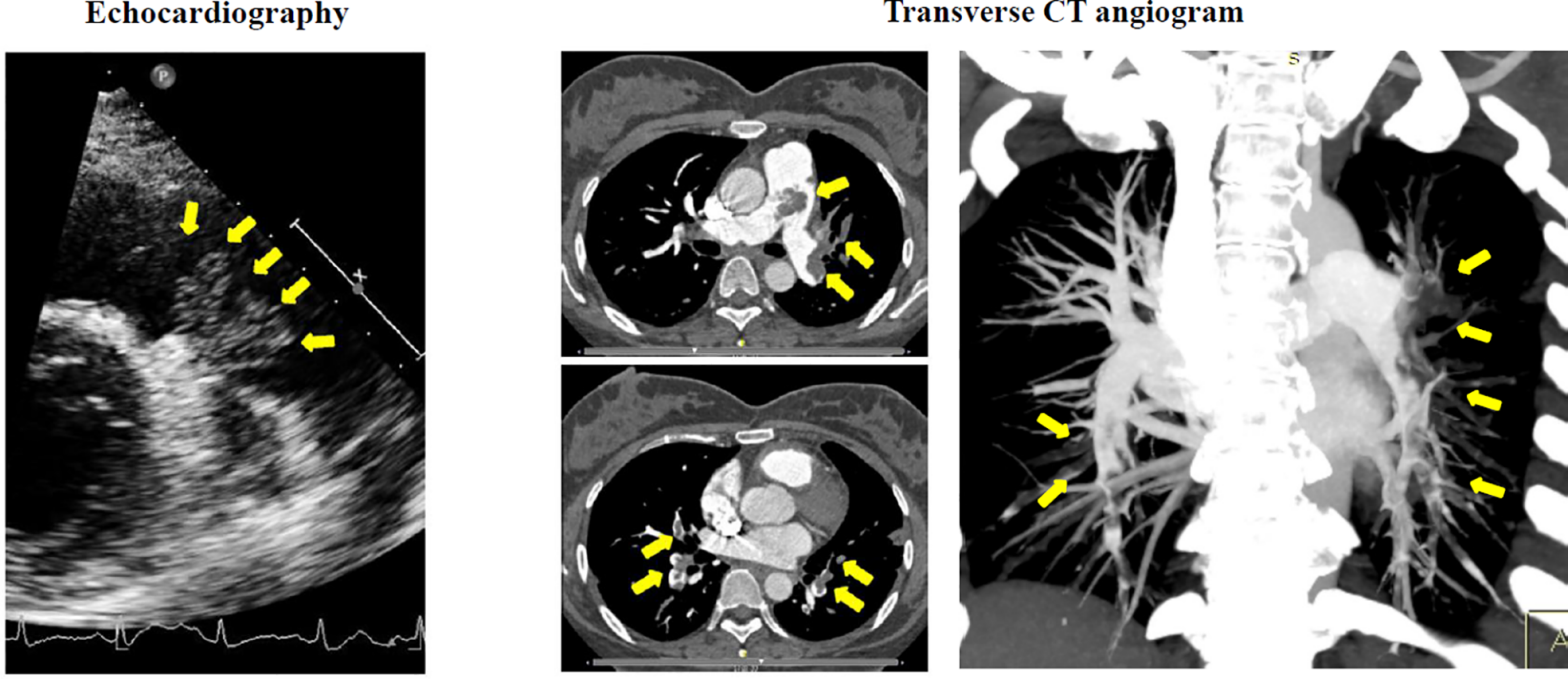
Preoperative echocardiography demonstrated a large lobulated mass (arrows) attached by a pedicle to the posterior wall of pulmonary truck. Transverse CT angiogram revealed a diffused occlusion of both pulmonary arteries (arrows).
